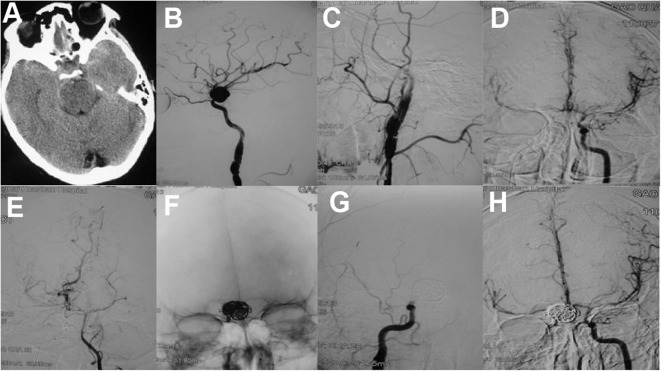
(A) An axial CT scan showed skull bone fracture and traumatic subarachonoid hemorrhage. (B) Two weeks later, the patient suffered rehemorrhage. A lateral cerebral angiogram of the right ICA demonstrated a large pseudoaneurysm at the C6 segment and a dissection of the C1 segment. Angiogram after balloon occlusion test (C) showed good compensation from the anterior communicating artery (D) and posterior communicating artery (E,F) The pseudoaneurysm and parent artery were trapped with six detachable coils. Postoperative right (G) and left (H) carotid angiograms showed exclusion of the pseudoaneurysm from the circulation [adapted from Lin et al. (72)].
